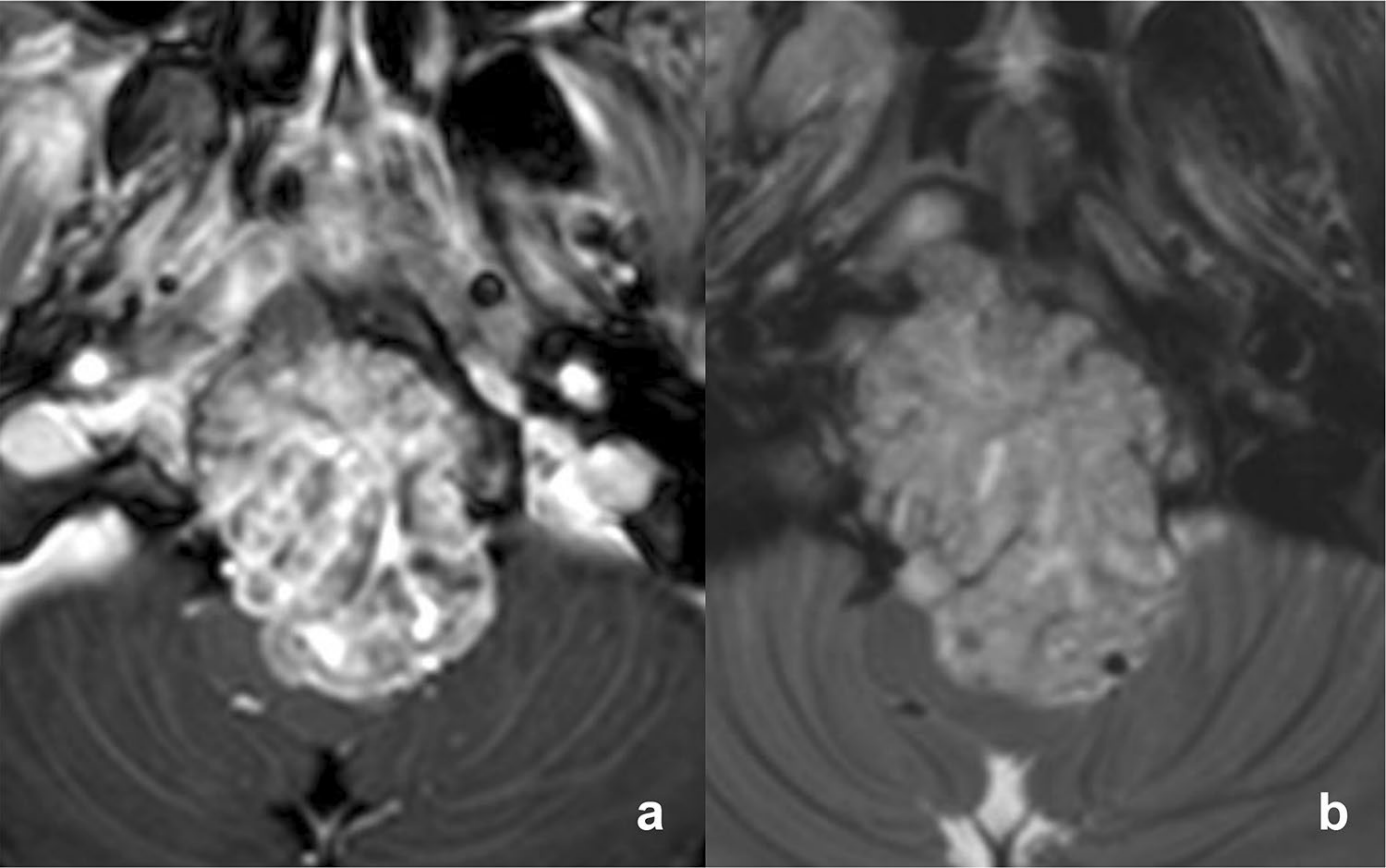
Magnetic resonance imaging of stroma-rich chordoma. (a) Contrast-enhanced T1-weighted and (b) T2-weighted imaging show an avidly enhancing expansile mass extending from the clivus with T2 intermediate signal intensity.
